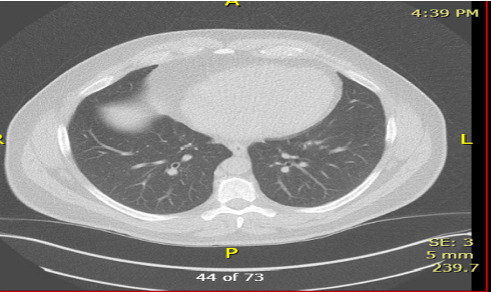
Chest CT scan findings—right lower lobe: enlargement of a previously identified nodule (now measuring 11 mm). Left upper lobe and right upper lobe: stable nodules (unchanged in size compared with prior imaging).
